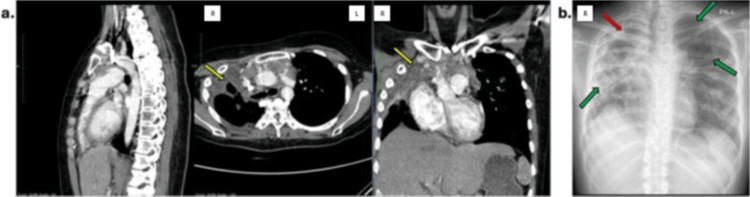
(a) Post-chemotherapy chest MSCT and (b) chest x-ray images revealed a 1.25 × 6.20 × 1.60-cm isodense, fine margin, and irregular lesion in the anterior mediastinum, consistent with a partial response of the mediastinal mass (yellow arrow); reactivated tuberculosis infection, evidenced by a cavitary lesion in the apex of the right lung (red arrow); and multiple hypodense, well-defined, thin-walled, air-filled lesions with regular margins observed bilaterally in the lungs, consistent with pulmonary bullae (green arrows). MSCT: Multislice computed tomography.
